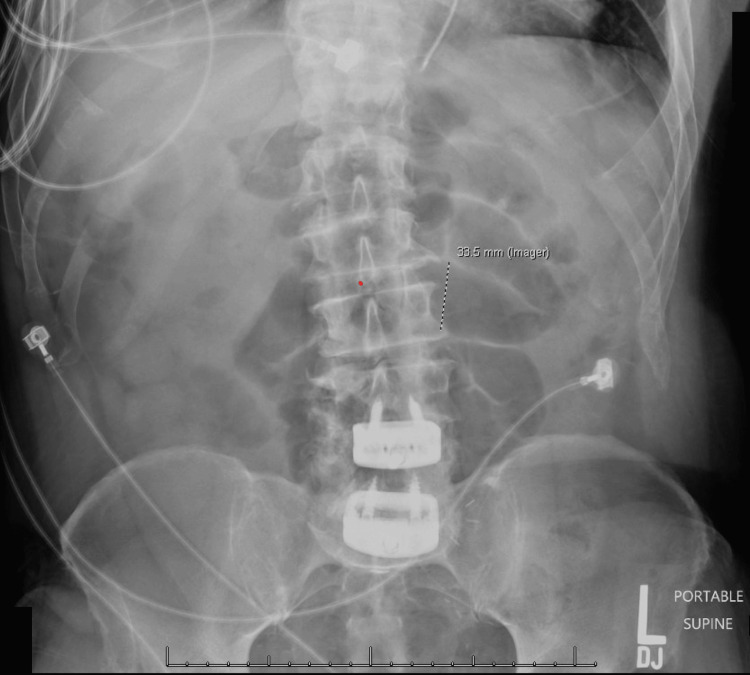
X-ray showing small bowel obstruction
